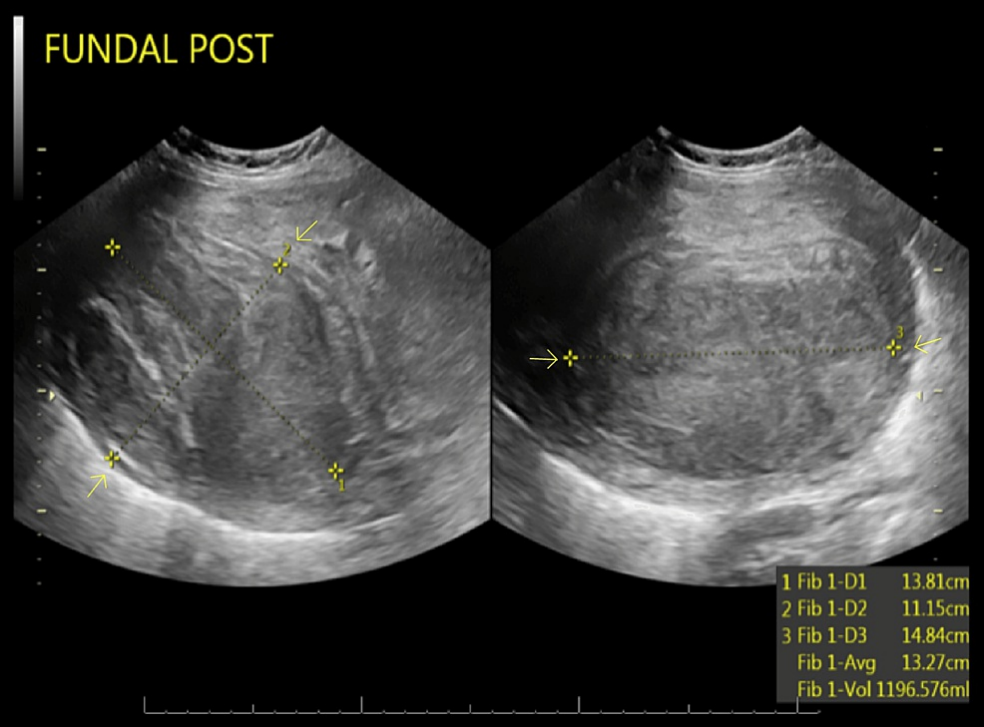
Second-trimester transvaginal ultrasound exhibiting sagittal and transverse views of the patients' largest fibroid (depicted by the yellow arrows)
